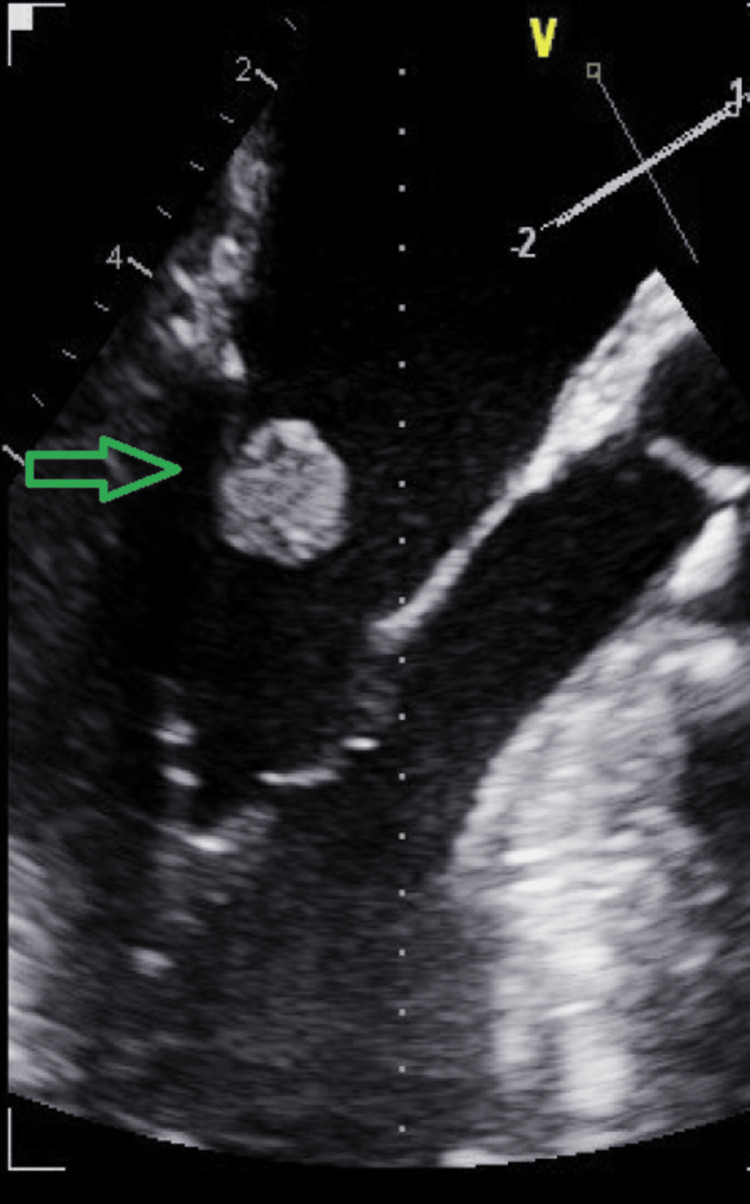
Transesophageal echocardiography showing a heterogeneous mass on the mitral valve.
